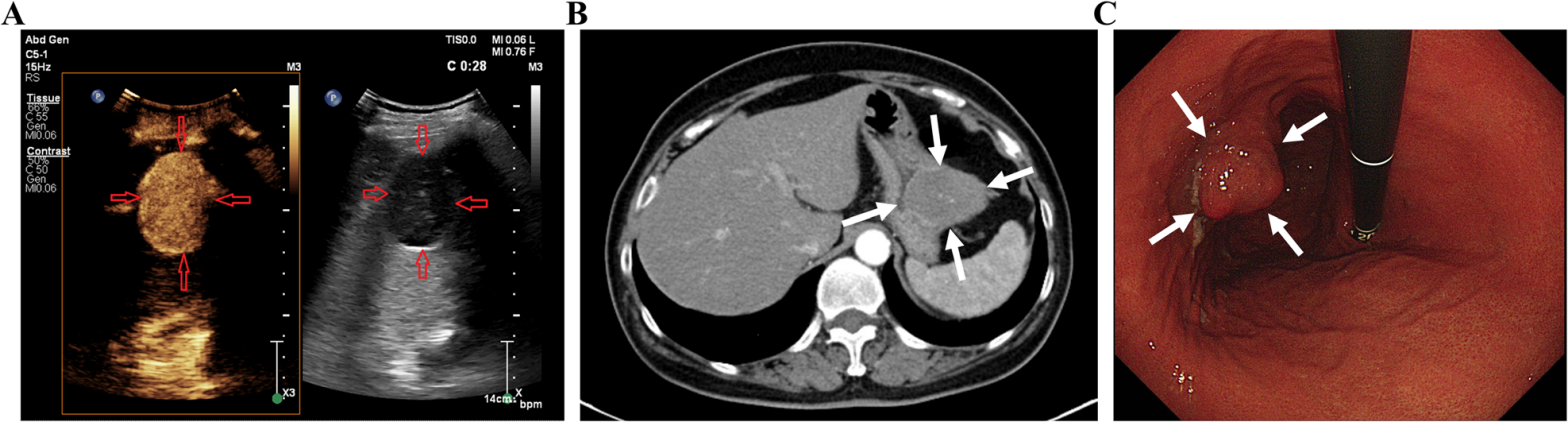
A An approx 65-year-old woman underwent gastric double contrast-enhanced ultrasonography and oral contrast ultrasonography, revealing a heterogeneous hyper-enhancement (left) and low echo under the gastric fundus mucosa(right), respectively, which was round and about 5.5 × 3.5 cm. The shape was regular with a smooth surface, and the boundary was clear. The hierarchical structure of the gastric wall was clear and not thickened. B Ellipsoid-shaped mass was seen on the greater curvature of the gastric body, with a size of about 56 × 38 mm, a clear boundary and partial lobes at the edge. After enhancement, it showed uniform and progressive enhancement on enhanced computed tomography. C Electronic gastroscopy found a diameter of about 3 cm submucosal bulge in the greater curvature side at the junction of the gastric fundus and the gastric body
